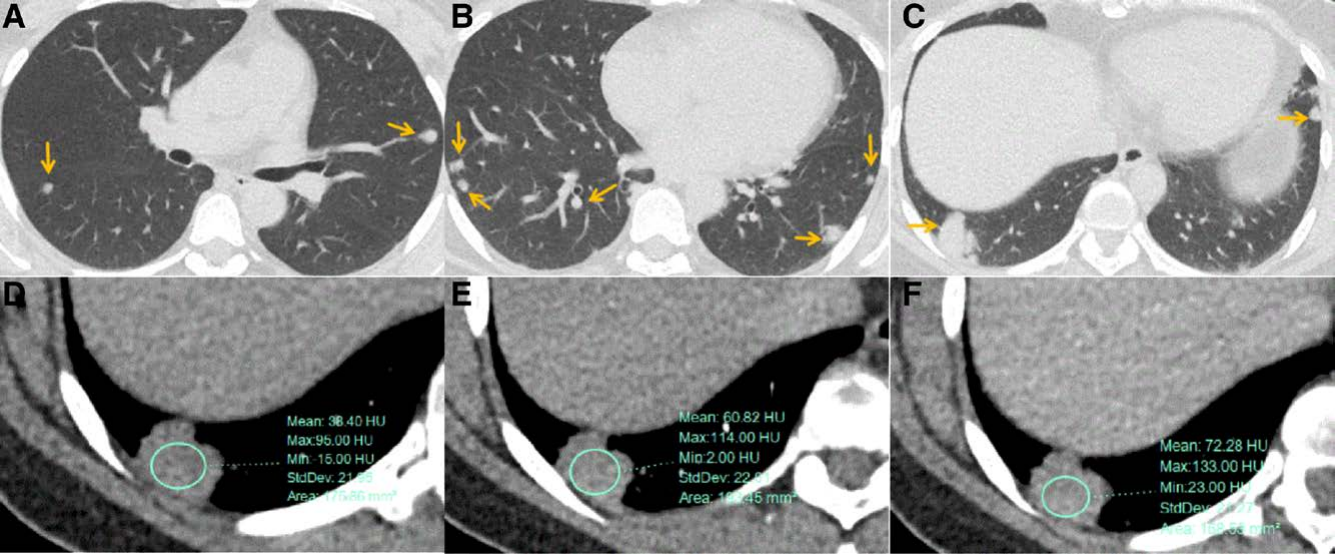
(A–C) Chest CT images showing multiple nodules in both lungs, the largest one is located in the right lower lung; (D–F) Enhancement scans of the largest lesion showing mild to moderate enhancement, which is less homogeneous. CT = computerized tomography.
